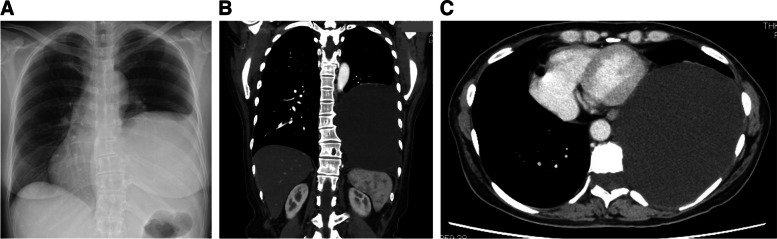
Preoperative chest X-ray image (a), and preoperative coronal (b) and axial (c) computed tomographic views showing the huge intrathoracic meningocele
